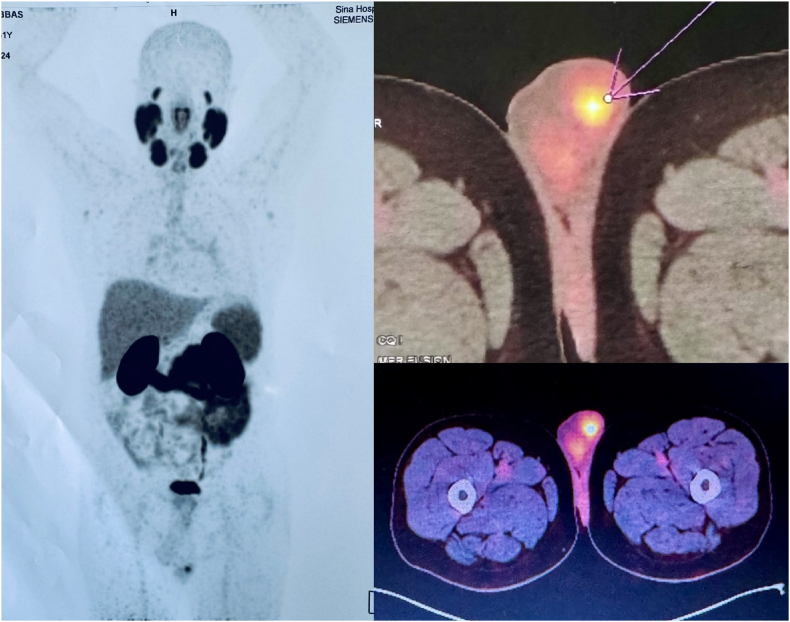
PET scan revealed focal increased Ga-PSMA uptake in the left scrotal region.
